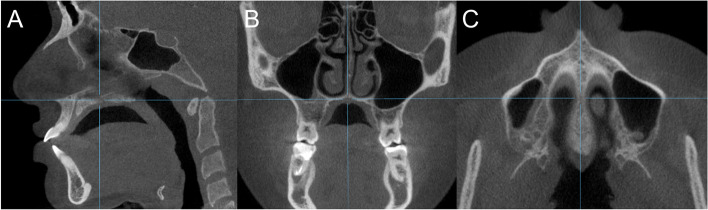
Orientation of the cone beam computed tomography scan in A Sagittal; B Coronal; and C Axial cuts
